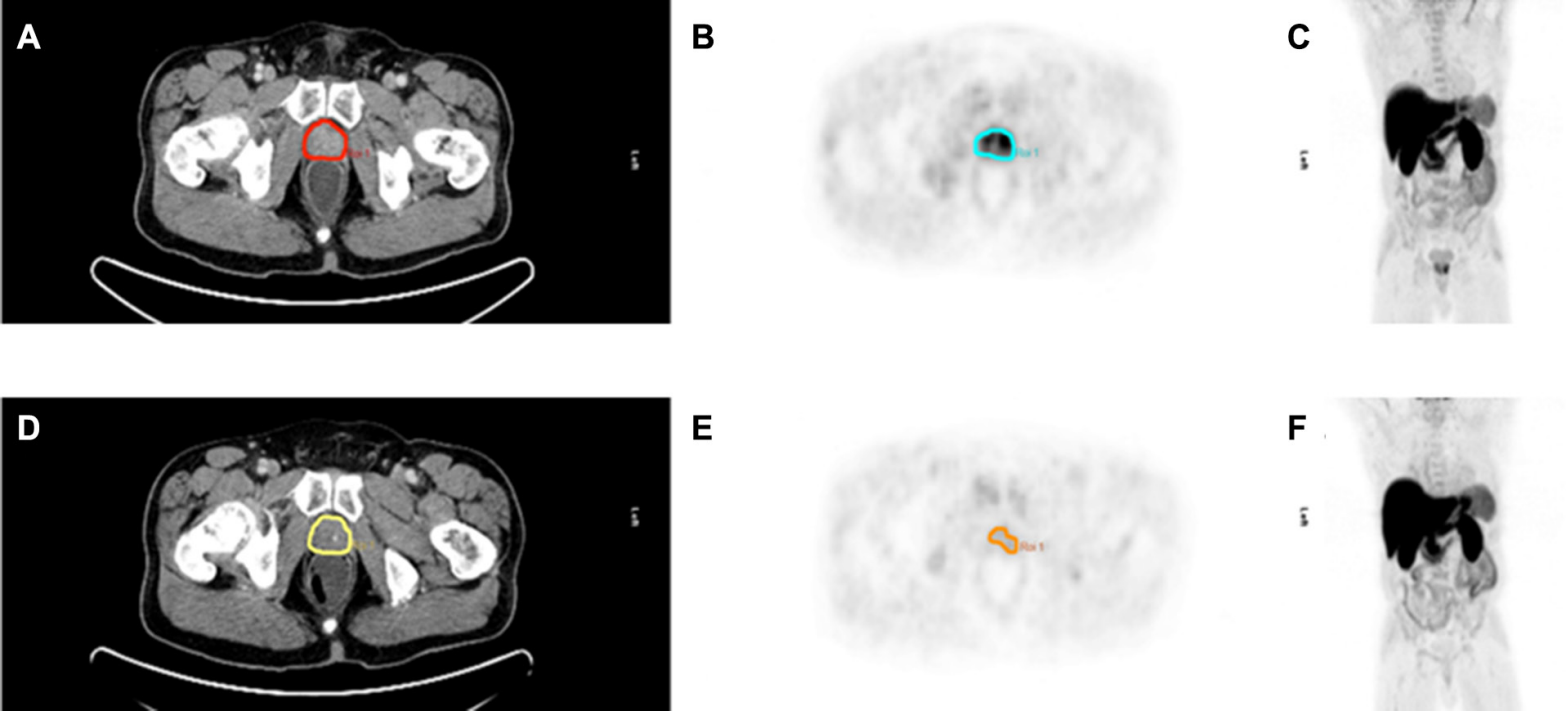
Pre- and posttherapeutic imaging example of a 66 year old patient with locally advanced prostate cancer (PSA before neoadjuvant therapy 12.63 ng/ml, Gleason score 6) before (A–C) and after neoadjuvant therapy (D–F) Significant decrease in choline uptake and prostate volume is shown in choline PET/CT after neoadjuvant therapy. PSA decreased down to 0.53 ng/ml after neoadjuvant therapy. A, B, C Pretherapeutic transaxial CT slice, PET slice, Maximum Intensity Projection (MIP) D, E, F
